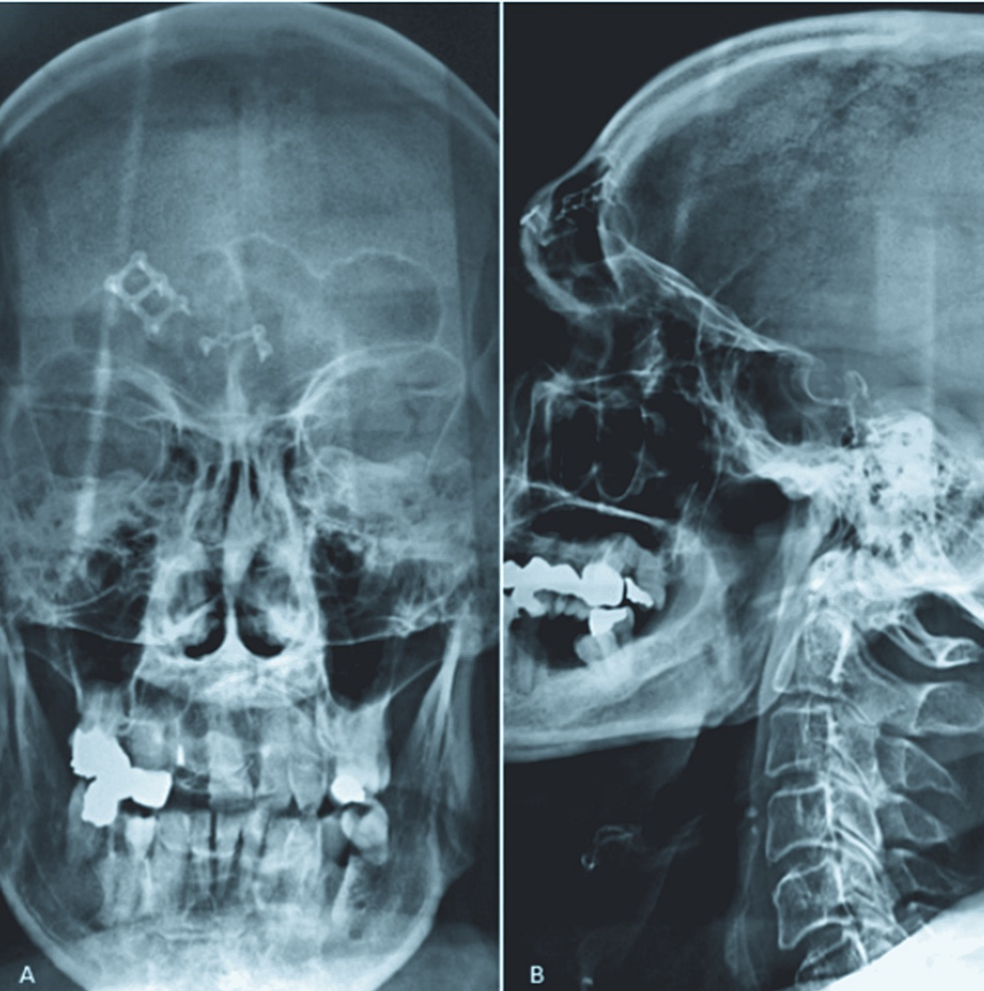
Post-operative x-ray showing the re-pneumatization of the right frontal sinus after six weeks of stenting of the nasofrontal duct (A: en face x-ray; B: profile x-ray).
